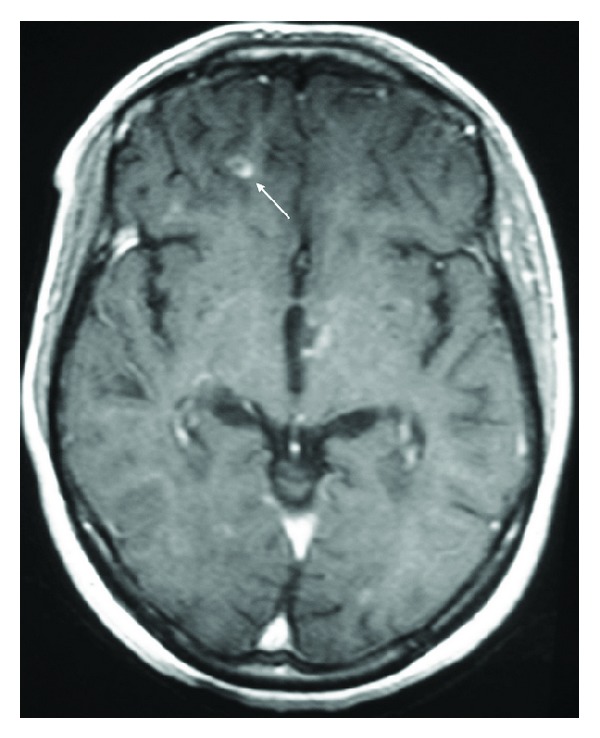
Axial cerebral T1-weighted image revealing a right orbitofrontal active lesion with gadolinium enhancement (arrow).
